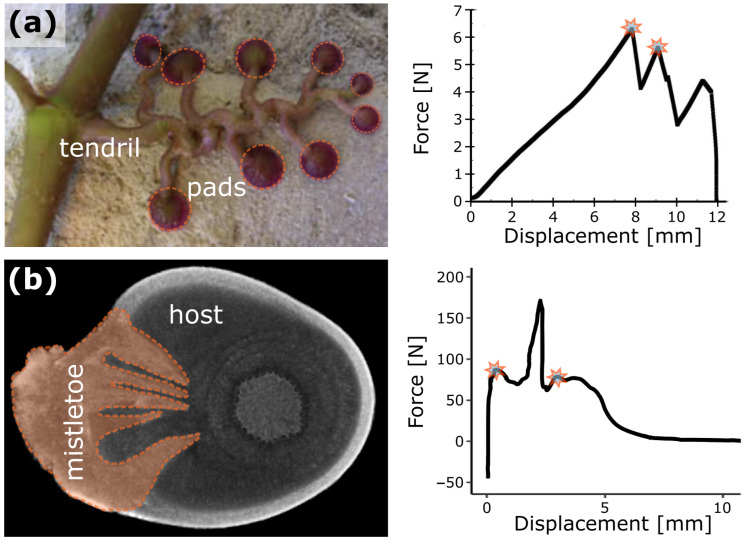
Hot redundancy mechanisms of biological material systems. (a) Adhesive tendril of Boston ivy (*Parthenocissus tricuspidata*) with nine redundant adhesive pads (circled in orange). The exemplary force-displacement curve shows stabilisation of the overall system after failure of individual pads (orange stars). Adapted with permission from [109]. (b) Cross-section of a micro-computed tomography image through the attachment site of a young mistletoe (*Viscum album*; coloured in transparent orange) and its host branch. Five wedge-shaped sinkers form the redundant mechanical and physiological connection between the two species. A force-displacement curve of a mistletoe–host sample under tensile load shows pre- and post-failure events, indicating the failure of individual sinkers (orange stars). Adapted from [110].
